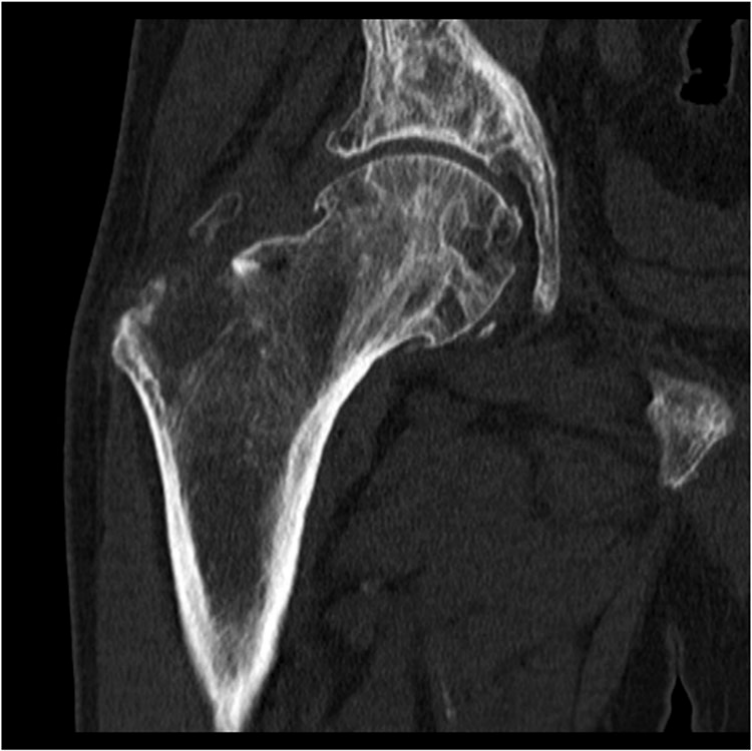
CT image of the right hip, showing a lytic and expanding lesion (Gaucheroma) of the greater trochanter, superiorly displacing fragments of bone
